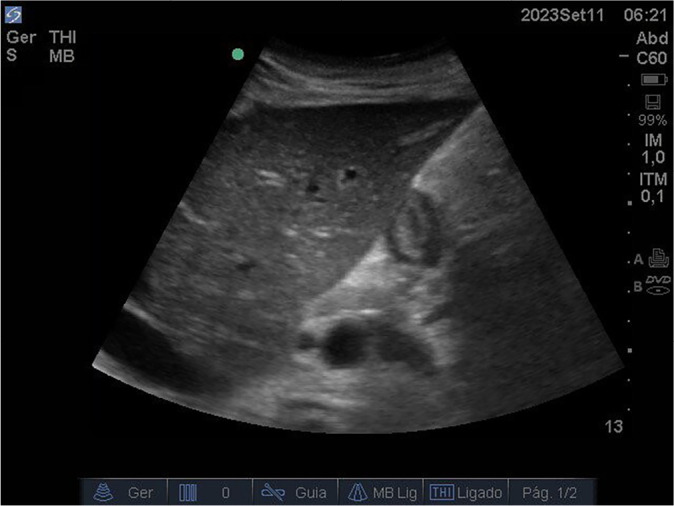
Gastric antrum ultrasound image on intensive care unit day 28
